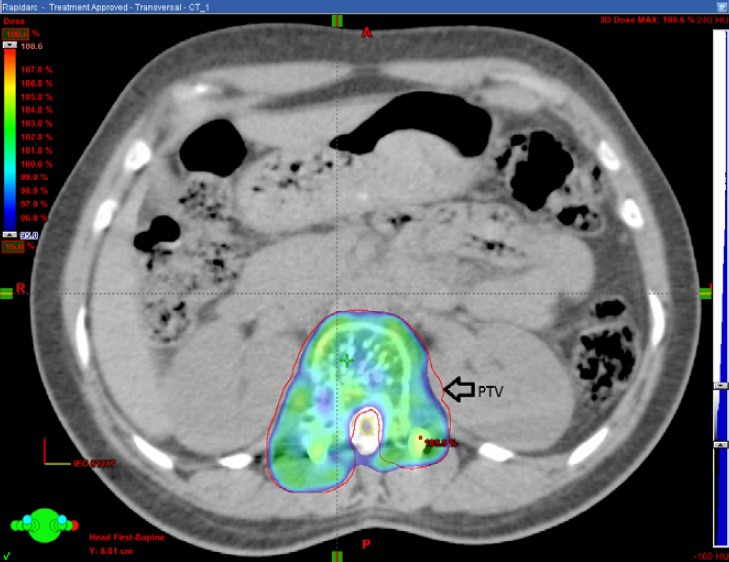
Outline of the PTV on CT myelogram.
